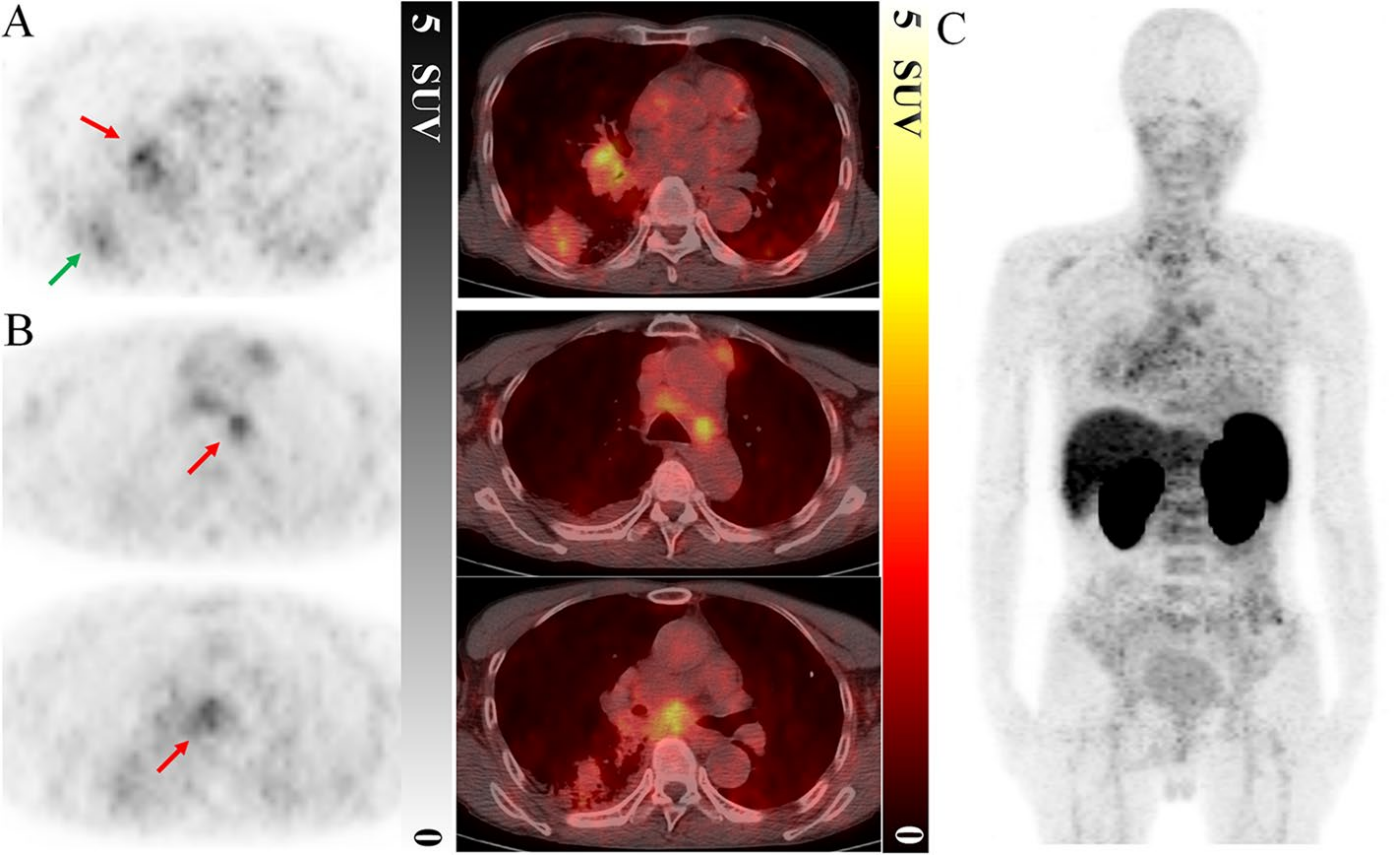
^68^Ga-NODAGA-NM-01 PET/CT of patient 1. Different uptake in the (A) right lung lower lobe primary lesion (green arrow), right hilar lymph node (red arrow), and (B) mediastinal lymph metastases. The SUV_max_ of primary lesion and metastases were 3.3, 4.4, and 3.6, respectively. C Representative maximum-intensity-projection image of patient 1
